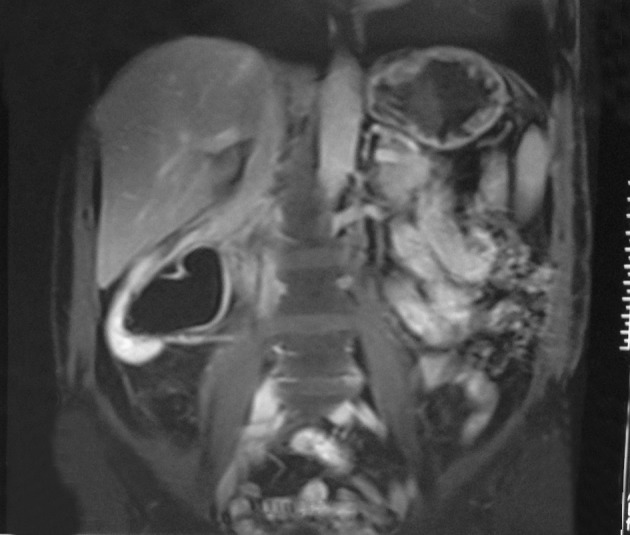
Coronal T1-weighted fat-suppressed contrast-enhanced MR image of the patient one month after surgery. There is no residual mass around the inferior vena cava.
